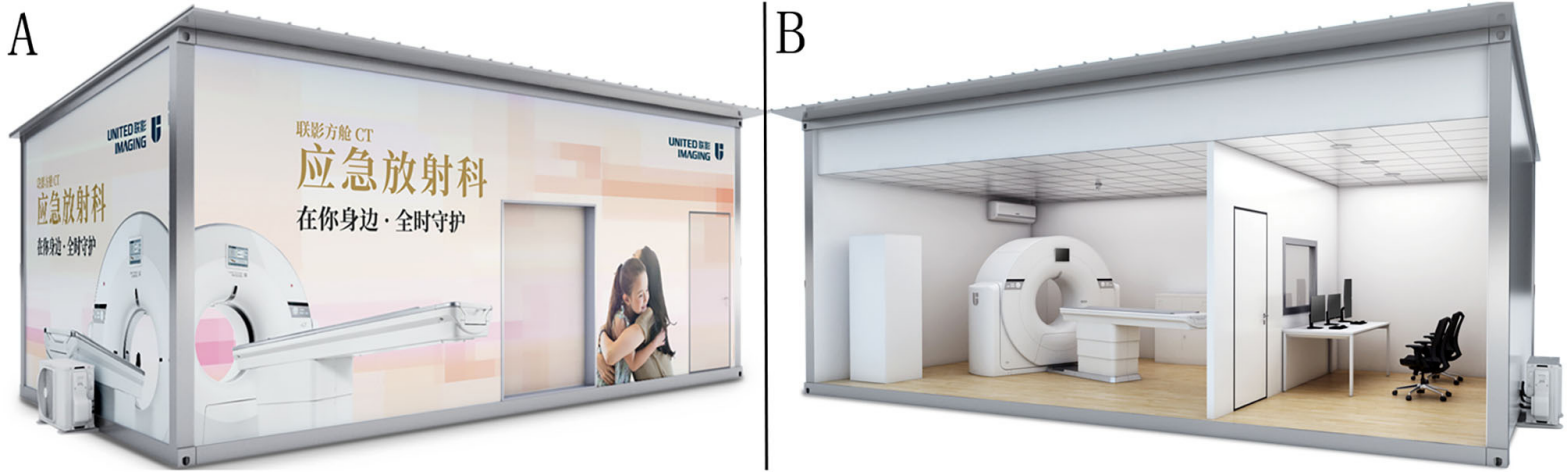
The structure of the relocatable CT system. (A) The outward appearance of the relocatable CT system. (B) The interior structure of the relocatable CT system. The figures were provided through the courtesy of Shanghai United Imaging Healthcare Co., Ltd.
